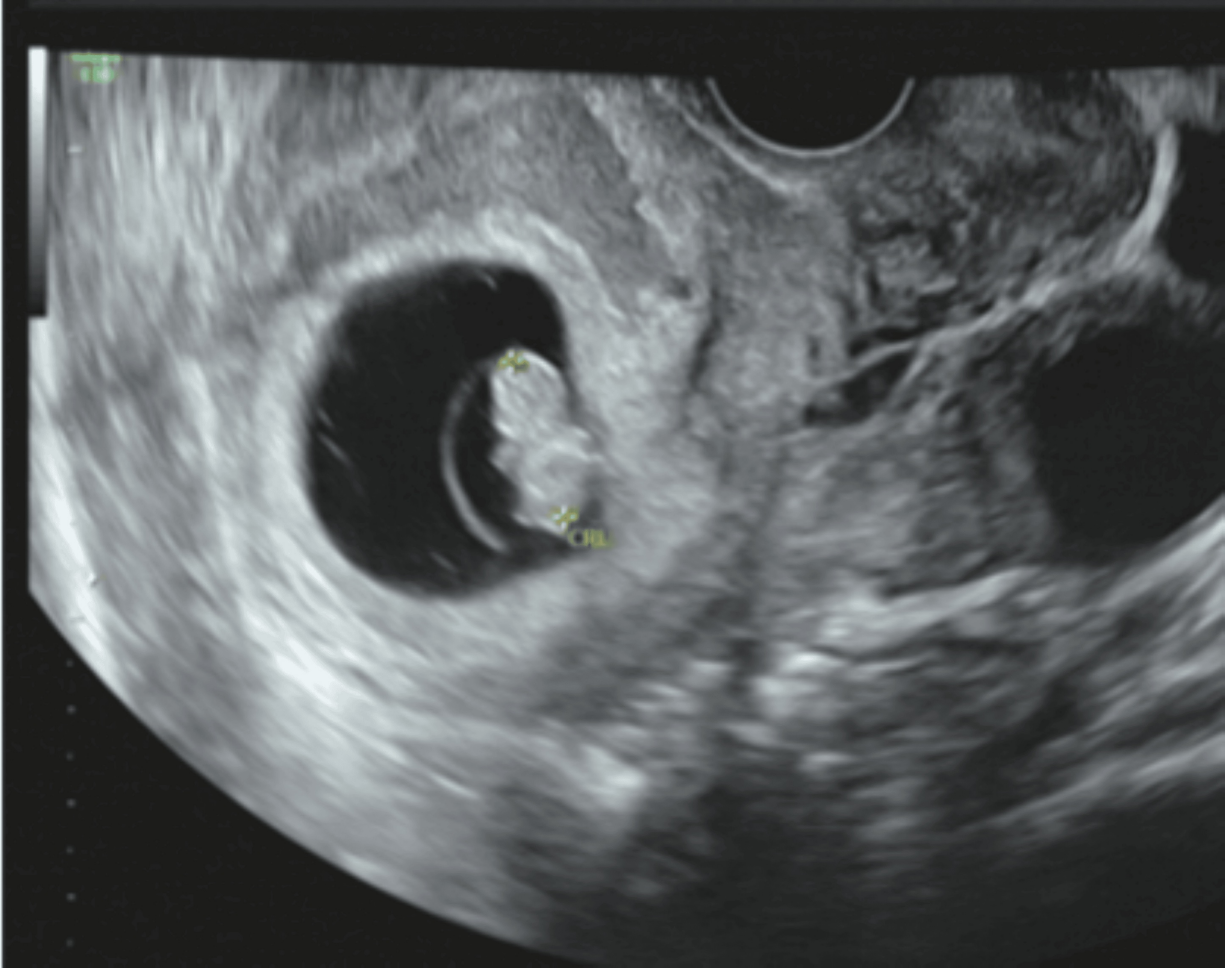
Transvaginal ultrasound. A fetus in the uterus.
